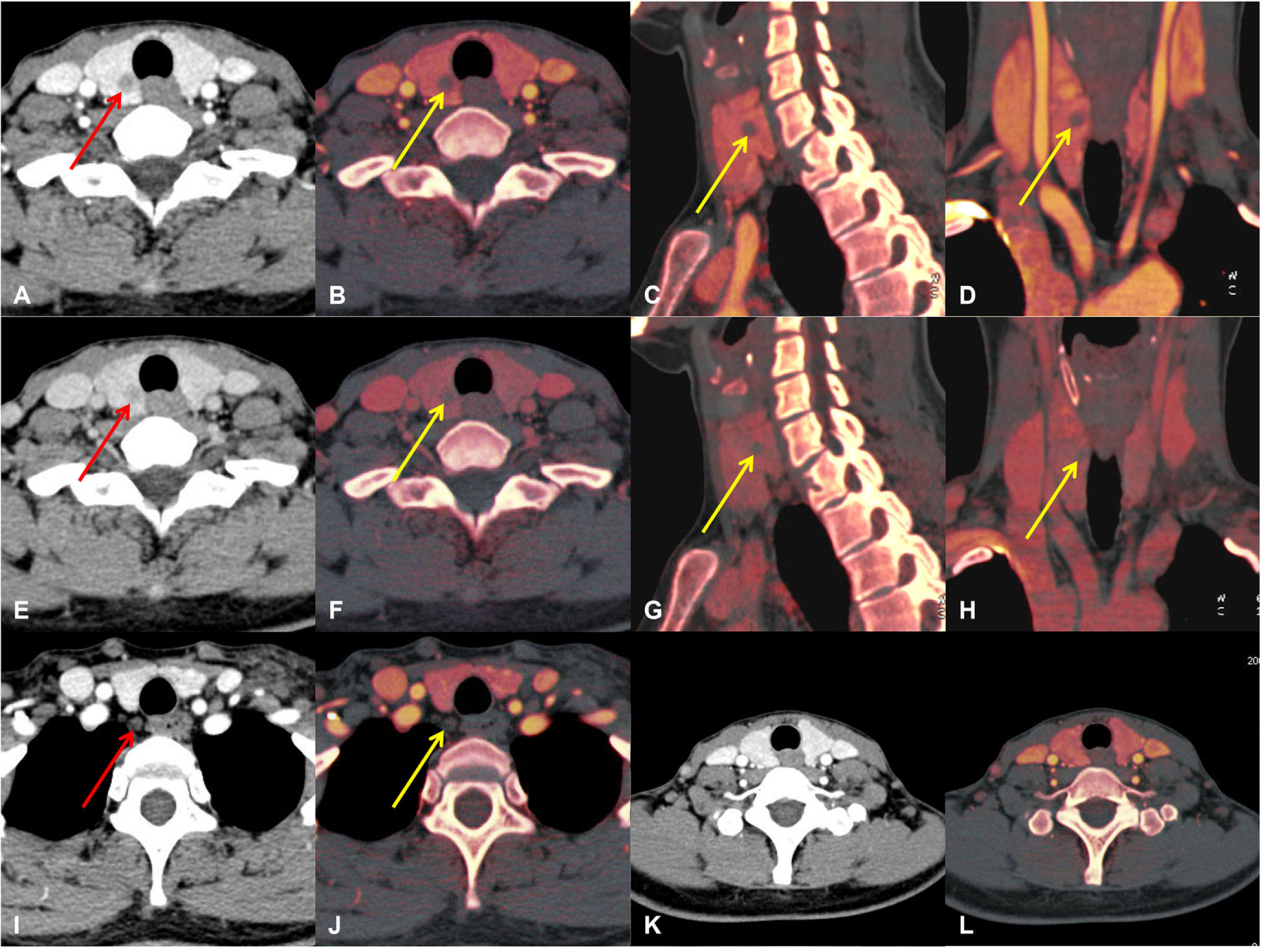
An example of a PTMC patient without LLNM. The right lobe and isthmus of the thyroid were excised, and lymph nodes in the right level VI were dissected. Postoperative pathology confirmed PTMC accompanied by HT, with a diameter of 0.9 cm, and no metastatic lymph nodes were found in the right level VI. a, e, A primary lesion located in the right lobe of thyroid on the contrast-enhanced arterial phase (a) and venous phase (e). b-d, The iodine maps of the primary lesion in the axial, sagittal, and coronal positions in the arterial phase (IC = 1.1 mg/mL). f-h, The iodine maps of the primary lesion in the axial, sagittal, and coronal positions in the venous phase (IC = 1.0 mg/mL). i, j, A benign lymph node located in the right level VIb on the arterial phase and iodine map. k, l, No metastatic lymph nodes were found in the lateral cervical region on the CT images. PTMC = papillary thyroid microcarcinoma, LLNM = lateral cervical lymph node metastasis, HT = Hashimoto’s thyroiditis, IC = iodine concentration, CT = computed tomography
